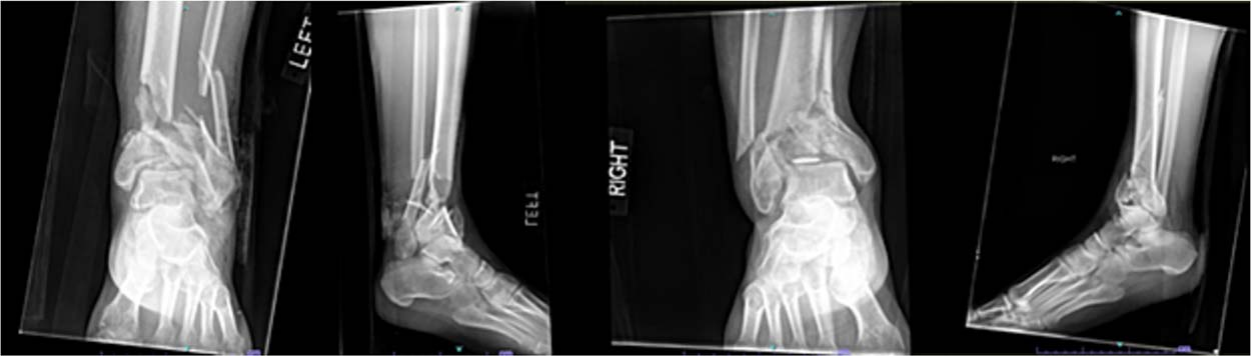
Initial AP and lateral radiographs in patient 3 demonstrating bilateral open pilon fractures with severe comminution of the left ankle.
